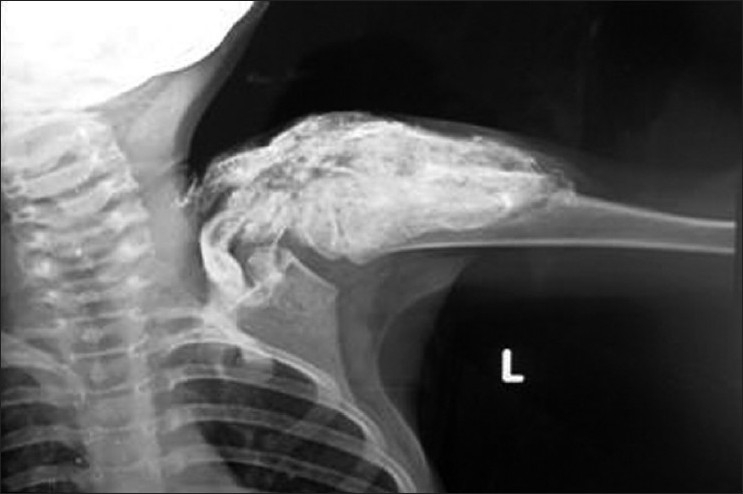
X-ray of left arm with shoulder joint anteroposterior view showing diffuse cocoon-like web of heterotopic bone
